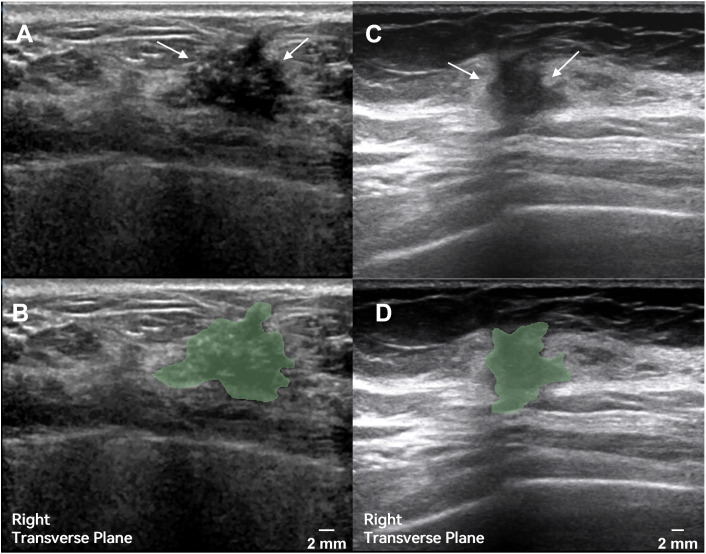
Representative ultrasound images of HER2-positive and HER2-negative tumors with ROIs drawn by ultrasonographers. A. Ultrasound image of a HER2-positive breast cancer patient. B. Corresponding ROI area for A. C. Ultrasound image of a HER2-negative breast cancer patient. D. Corresponding ROI area for C. Arrows in A and C indicate the tumor regions; the green areas in B and D represent tumor masking regions, annotated by two physicians in a double-blind manner. All images are cross-sectional views of the right breast, scale bar of 2 mm.
